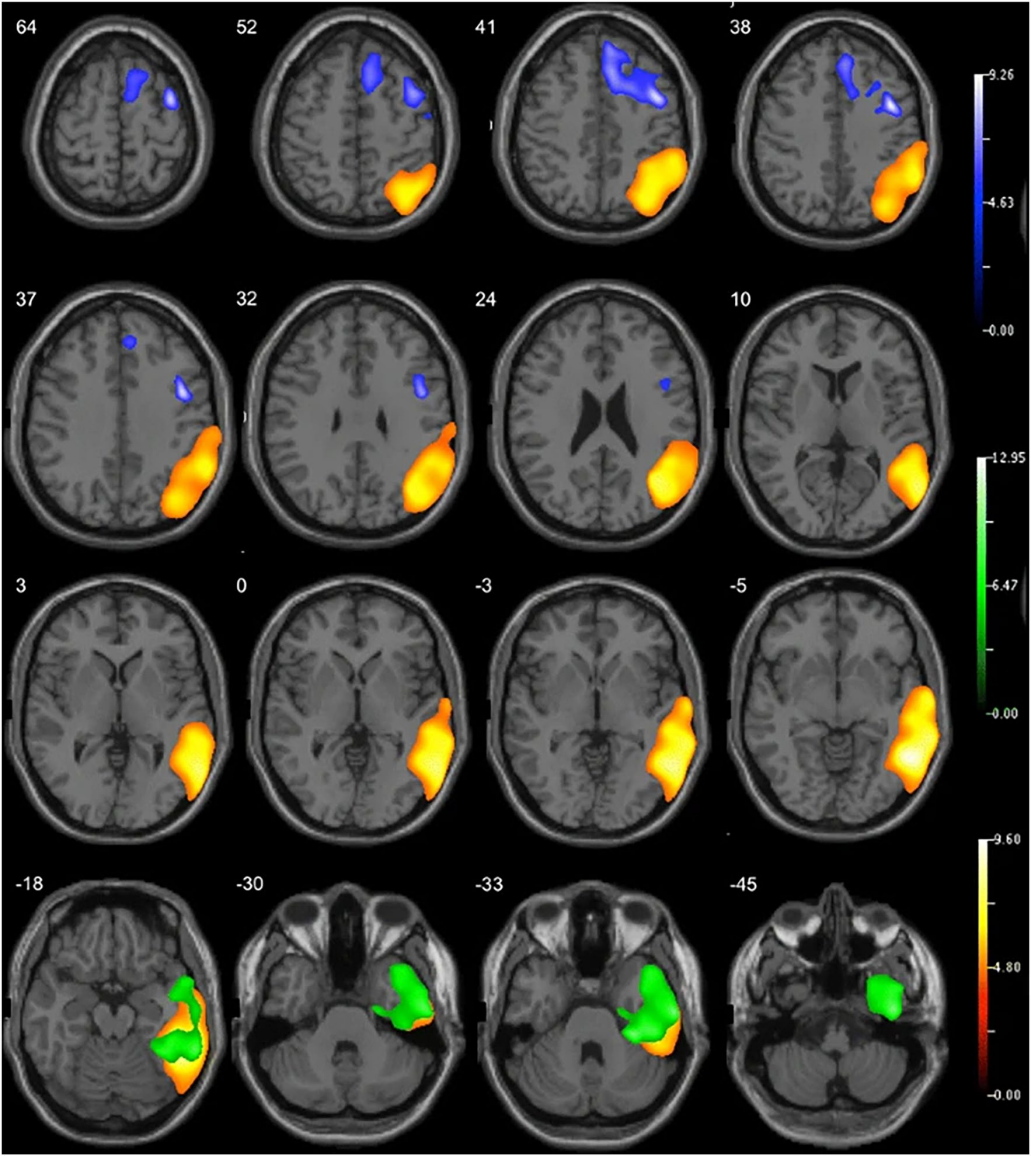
Delineation of hypometabolic regions at [^18^F] fluorodeoxyglucose positron emission tomography (FDG-PET) which can aid in differentiation subtypes of primary progressive aphasia. The blue areas indicate the nonfluent/agrammatic subtype, green indicates the semantic subtype, and yellow represents the logopenic subtype. Reproduced with permission from Matías-Guiu et al. [19]
